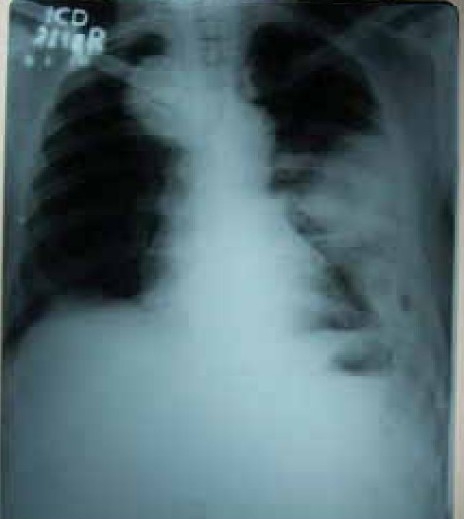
Chest X-ray post thoracoscopy
